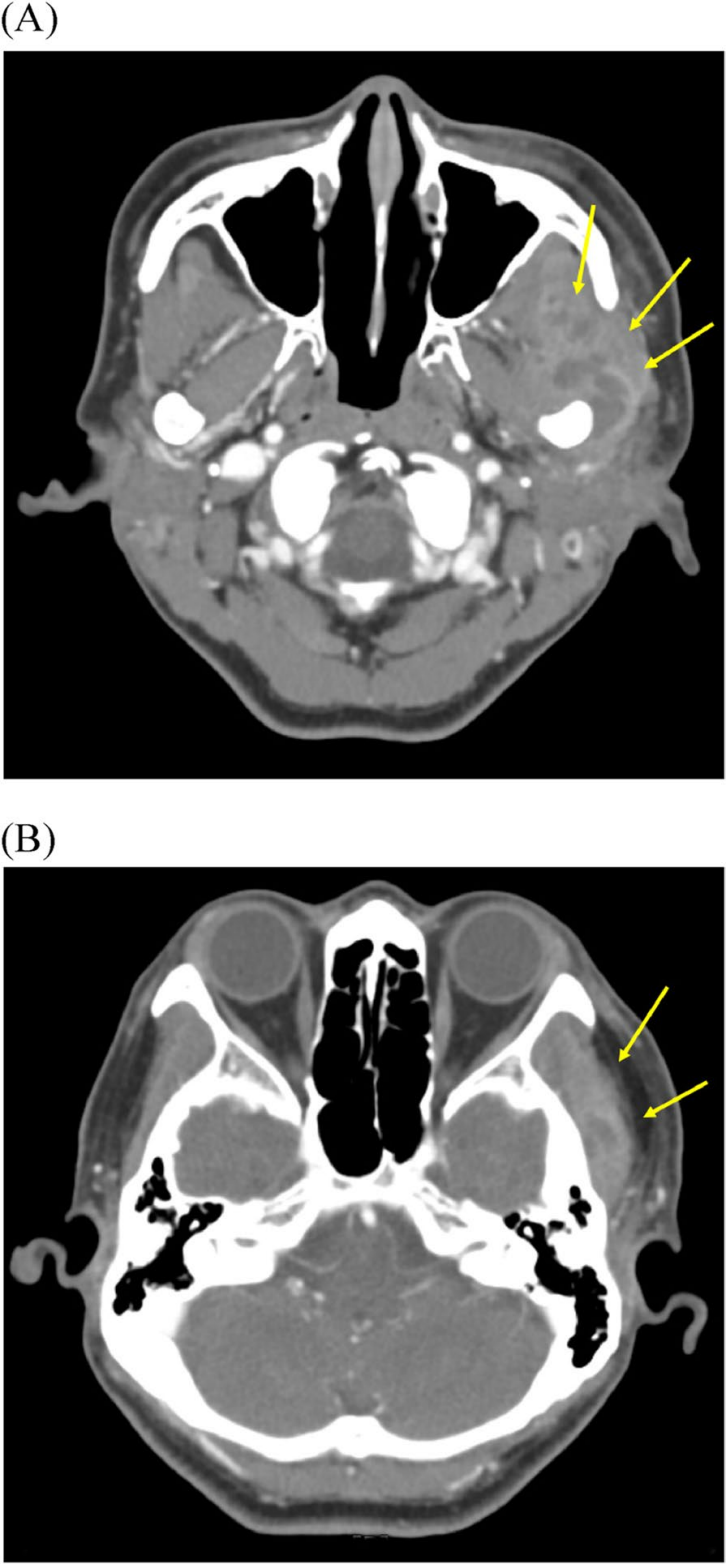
Enhanced CT images at first visit (case 1). Heterogeneous lesions of left masseteric and temporal space (yellow arrows) were observed. A Masseteric space. B Temporal space
